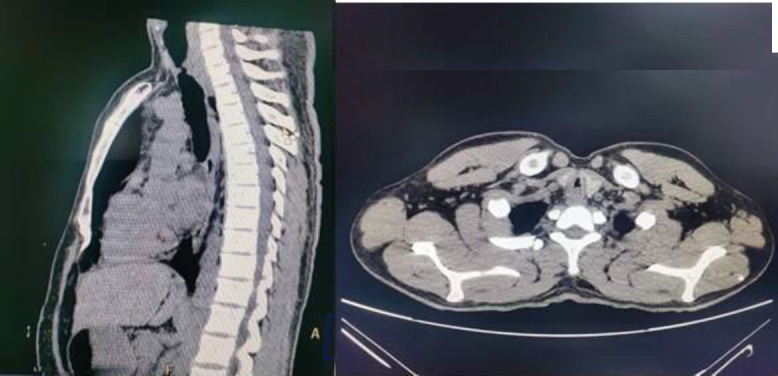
CT scan images showing tracheal stenosis
